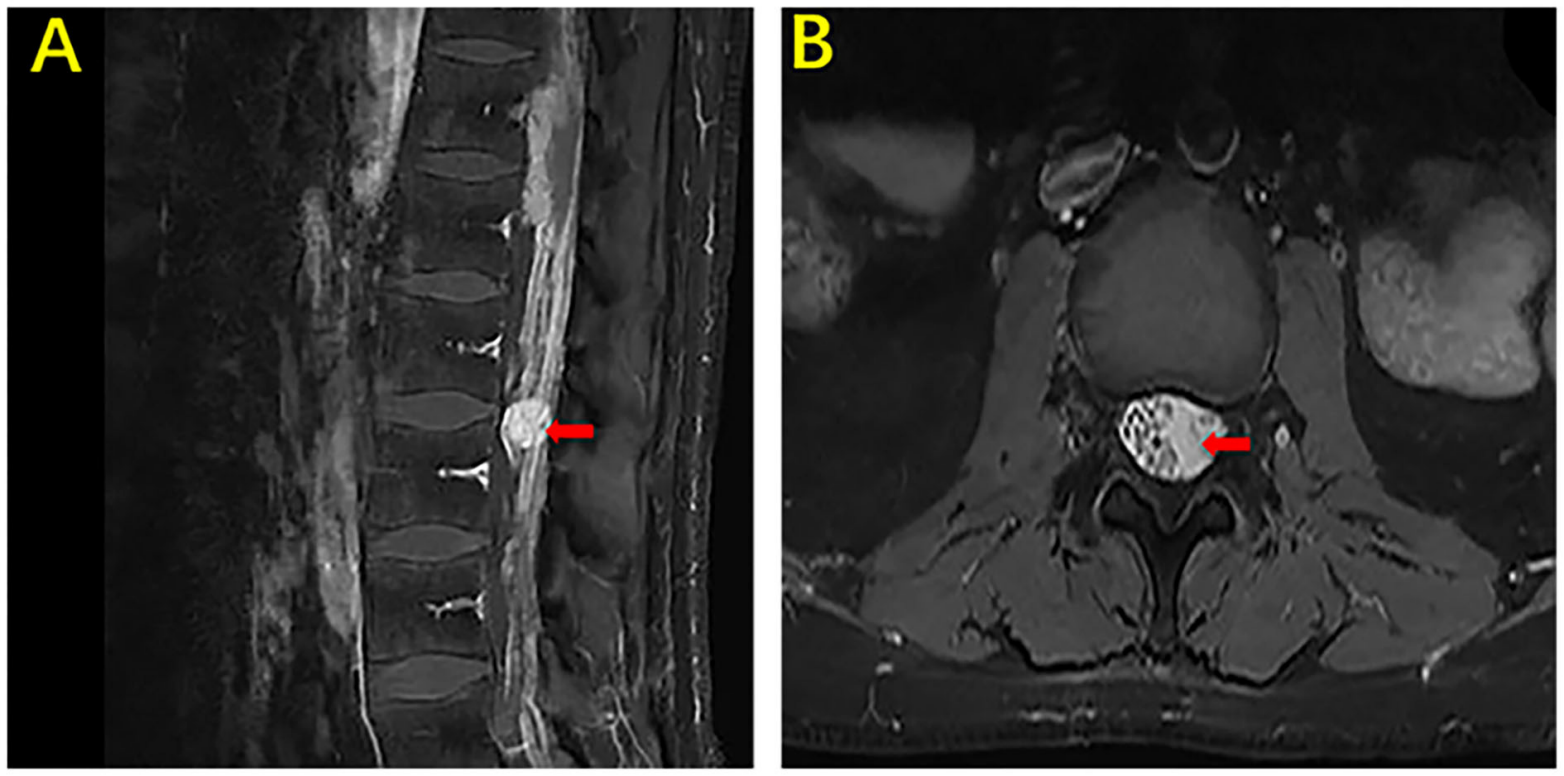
MRI of the brain and lumbar vertebra of our case. The sagittal section and axial section of the lumbar vertebra showing marked nodular enhancement in the spinal cord at the level of the second lumbar vertebra (arrow) (A). The axial section of the lumbar vertebra showing marked nodular enhancement in the spinal cord at the same level in picture (A) (arrow) (B).
